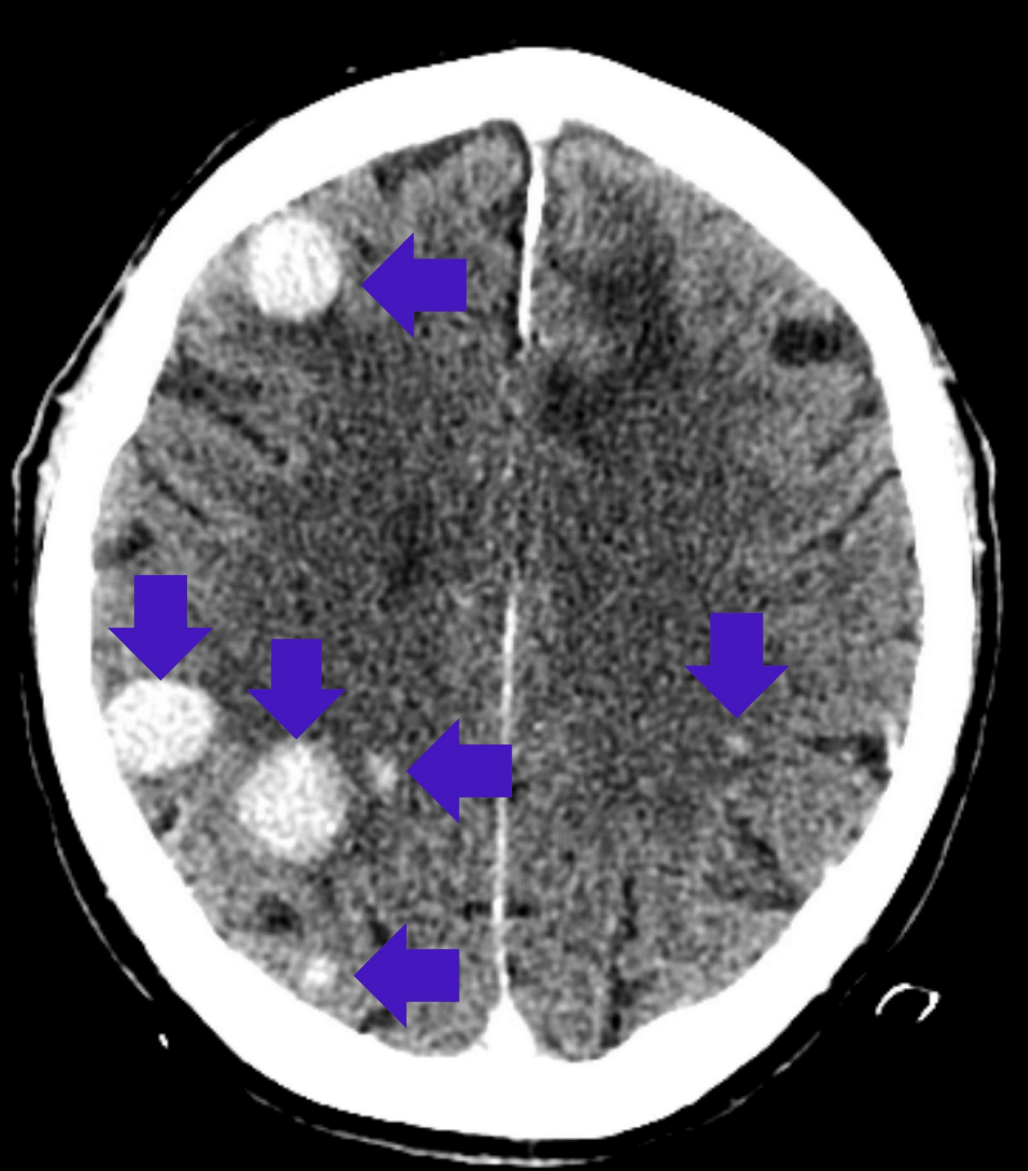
Contrast-enhanced head CT of patient 3 with SCNSL There are numerous hyperdense mass lesions in the bilateral cerebral hemispheres with contrast enhancement. SCNSL = secondary central nervous system lymphoma
